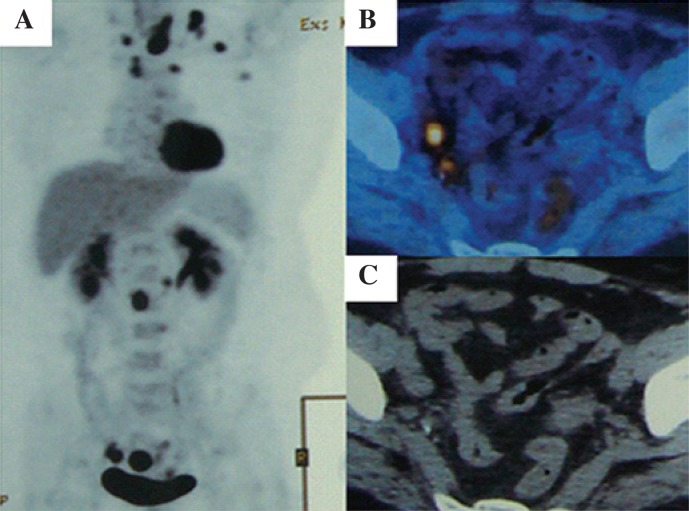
Whole body positron emission tomography (PET) scan combined with computed tomography (CT). Coronal maximum intensity projection image revealing (A) intense FDG uptake in the bilateral supraclavicular lymph nodes. PET/CT image (B) and CT image (C) revealing an enlarged right ovary lesion with intense FDG uptake. FDG, ^18^F-fluorodeoxyglucose.
